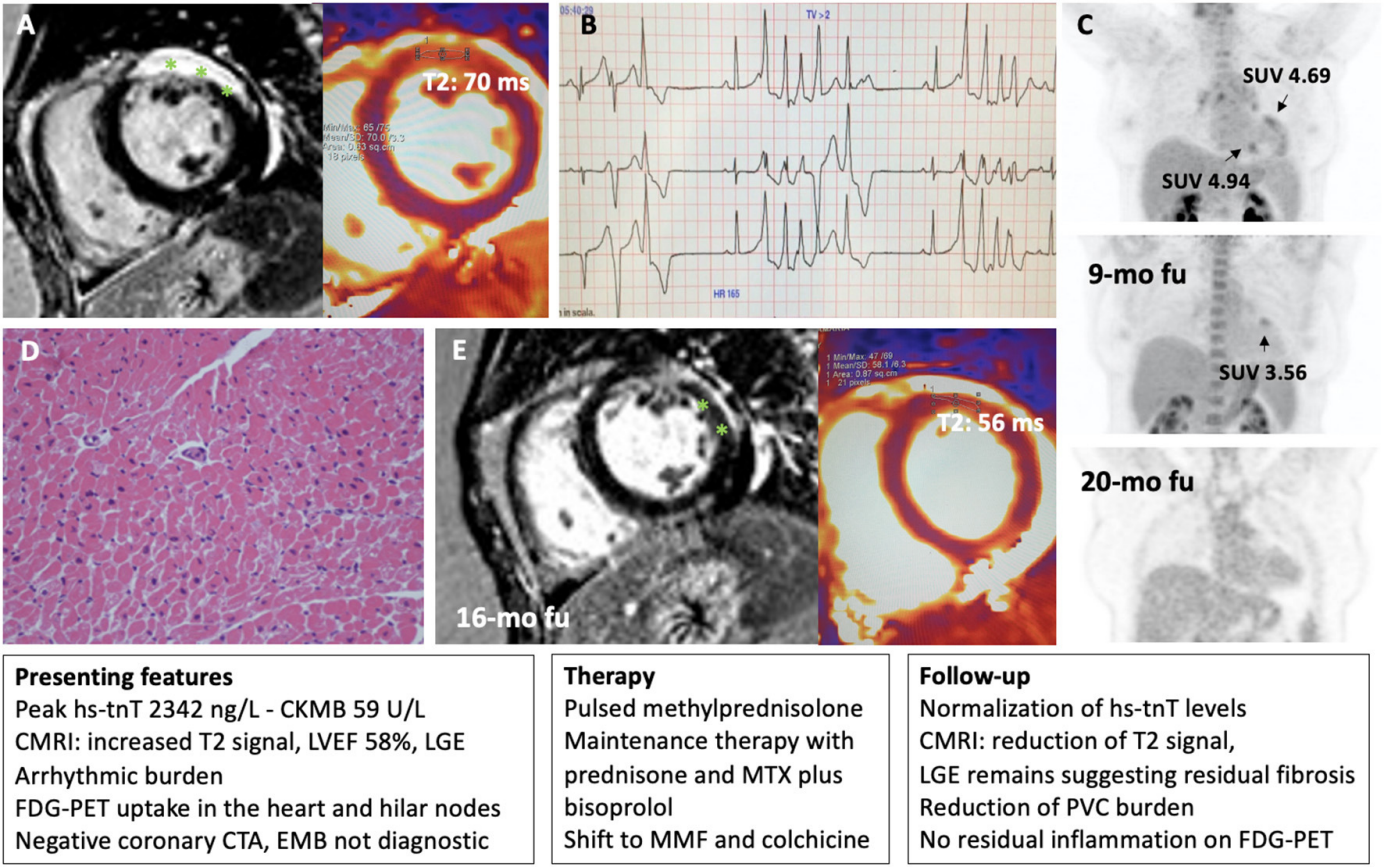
Representative patient with acute myocarditis in whom immunosuppression was guided by FDG-PET and cardiac magnetic resonance imaging (CMRI). A 49-year-old woman with a previous history of ANA positive pericarditis presented with acute myocarditis. On CMRI she presented a transmural lesion in the anterior wall as demonstrated by late gadolinium enhancement (LGE, highlighted with asterisks) and increased T2 signal (normal value <55 ms) (A). Left ventricular ejection fraction (LVEF) remain preserved, but a ventricular arrhythmic burden was observed on telemetry monitoring with frequent premature ventricular complexes (PVC) and non-sustained ventricular tachycardia (NSVT) (B). An FDG-PET showed 3 focal areas of uptake in the heart with increased standardized uptake values (SUV), and an uptake in hilar nodes raising the suspect for cardiac sarcoidosis (C). A septal endomyocardial biopsy (EMB) from the right ventricle was non-diagnostic for myocarditis or cardiac sarcoidosis (D). Peak high sensitivity troponin T (hs-tnT) levels was 2,342 ng/L. After initial pulsed methylprednisolone, prednisone was started in combination with methotrexate (MTX) and later shift to mycophenolate mofetil (MMF) and colchicine plus bisoprolol with normalization of troponin levels, and no signs of residual inflammation on FDG-PET (C), and a reduction of PVC burden. Accordingly, CMRI showed a reduction of T2 signal while LGE remains suggesting an area of residual fibrosis (E).
